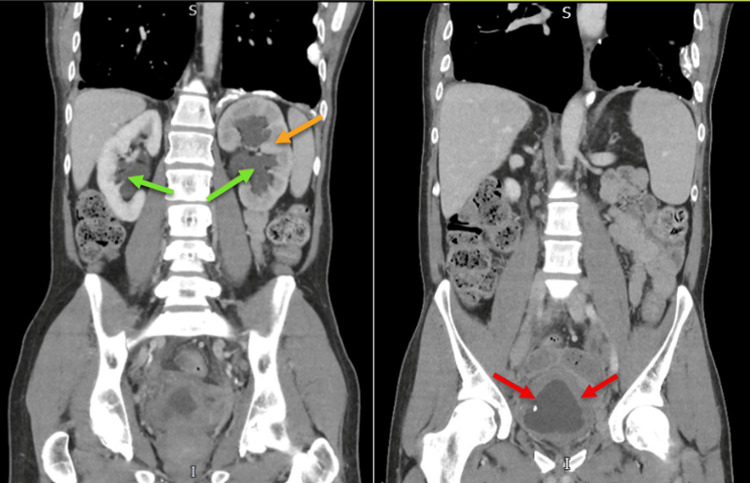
Coronal contrast CT TAP at initial presentation Bilateral hydronephrosis and pelvicalyceal dilatation can be seen (green arrows). The left kidney shows reduced parenchymal enhancement compared to the right, consistent with pyelonephritis (orange arrow). The bladder is visible inferiorly and shows diffuse wall thickening with a 6 mm calcification (red arrows). CT TAP: computed tomography of the thorax, abdomen, and pelvis
